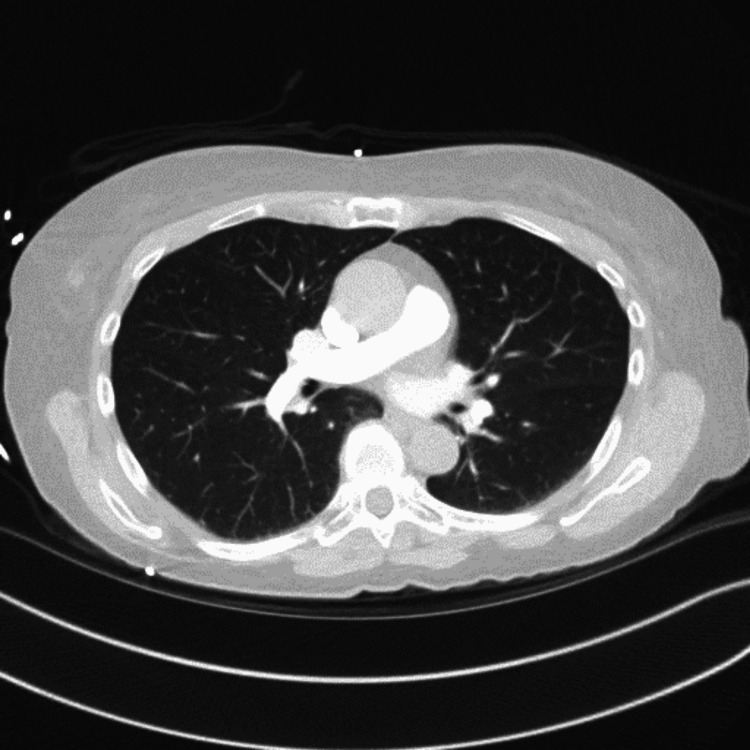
An image from an axial computed tomography (CT) angiography pulmonary embolism protocol conducted on the day of presentation to the Emergency Department, showing no evidence of pulmonary embolism or acute cardiopulmonary abnormalities.
